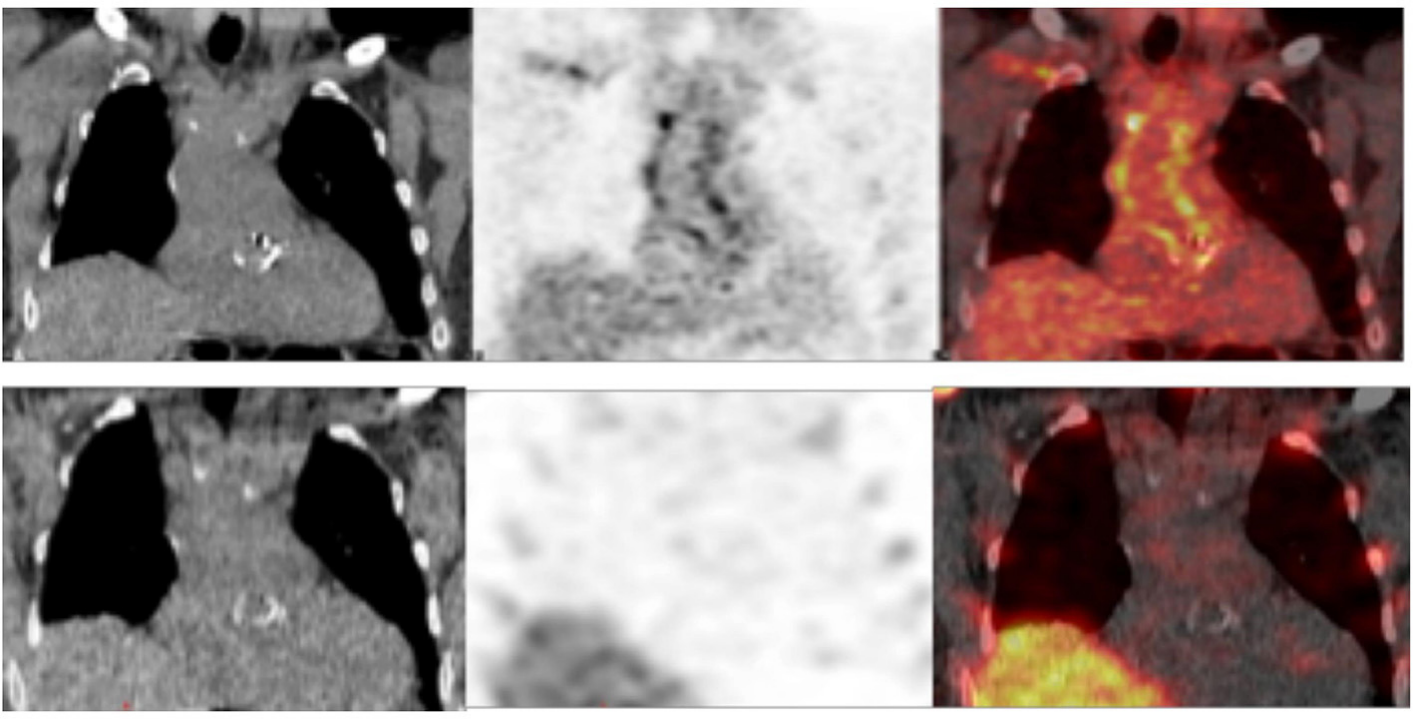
Examples of discordant findings between [^18^F]FDG PET/CT (upper panel, coronal view from left to right CT, emission and superimposed PET/CT) and ^99m^Tc labeled hexamethylpropylene amine oxime-WBC (^99m^Tc-HMPAO-WBC) (lower panel, coronal view from left to right CT, emission and superimposed SPET/CT) in a patient imaged 1 month after the Bentall procedure. PET/CT showed increased [^18^F]FDG uptake at both the aortic valve and the vascular graft (diffuse pattern with areas of focal accumulation), while WBC SPECT/CT results negative. A 2-year follow-up excluded infection.
